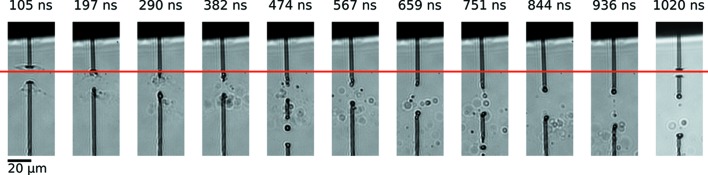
Sideview images showing the arrival of the second X-ray pulse in a train of pulses. The water jet was operated under the same conditions described in Fig. 4 ▸. The horizontal red line indicates that the jet has recovered and is stable in the X-ray interaction region after <290 ns. The last image in the sequence shows the jet after being hit by the second pulse in the pulse train. The scale bar is 20 µm. X-rays are incident from the left.
